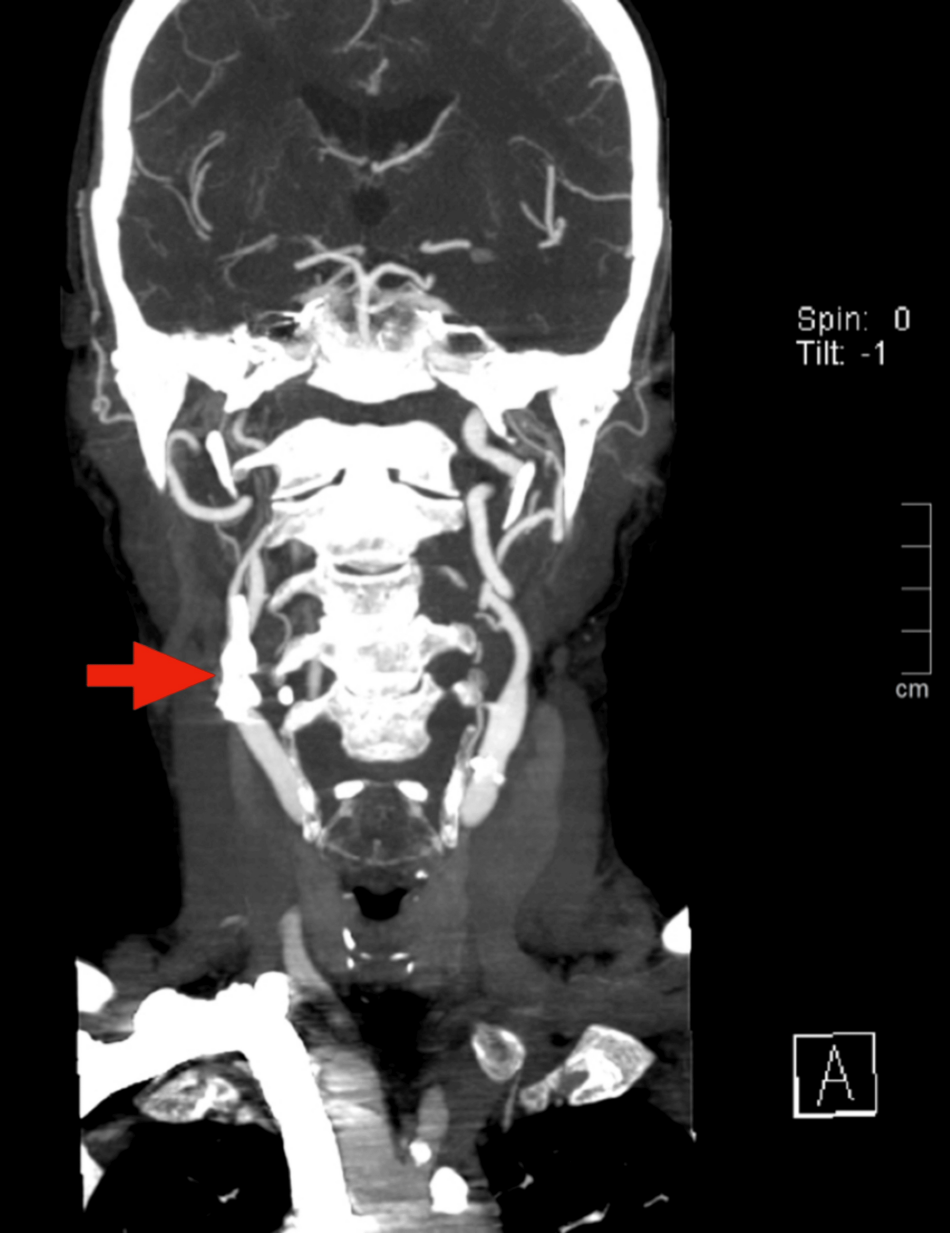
Coronal cervicoencephalic CT angiography shows calcified atherosclerotic plaques in the right carotid artery
